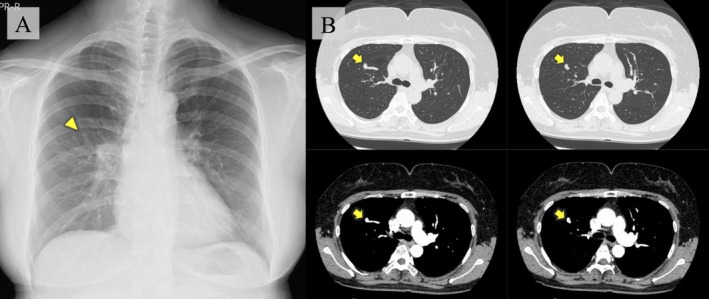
(A) Chest radiograph showing a small nodule in the right middle lung field with well‐defined margins in continuity with the blood vessels (triangle). (B) Contrast‐enhanced chest computed tomography showing an abnormal vascular picture, suspicious for pulmonary arteriovenous malformation (arrow).
